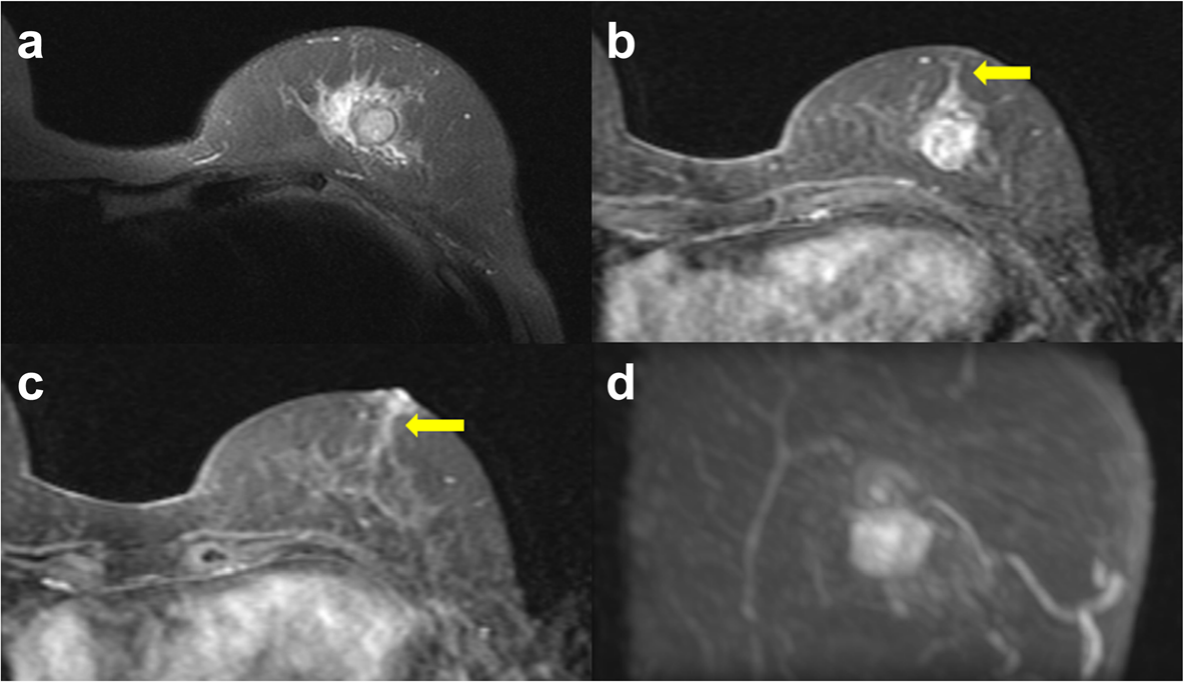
a Magnetic resonance imaging (MRI) shows that the mass has a relatively high intensity and has a peritumoral low-intensity capsule-like signal on T2-weighted images. b, c Contrast-enhanced MRI shows a mass with early arterial enhancement and two areas of linear enhancement between the mass and nipple (*arrows*). d Maximum intensity projection (MIP)
